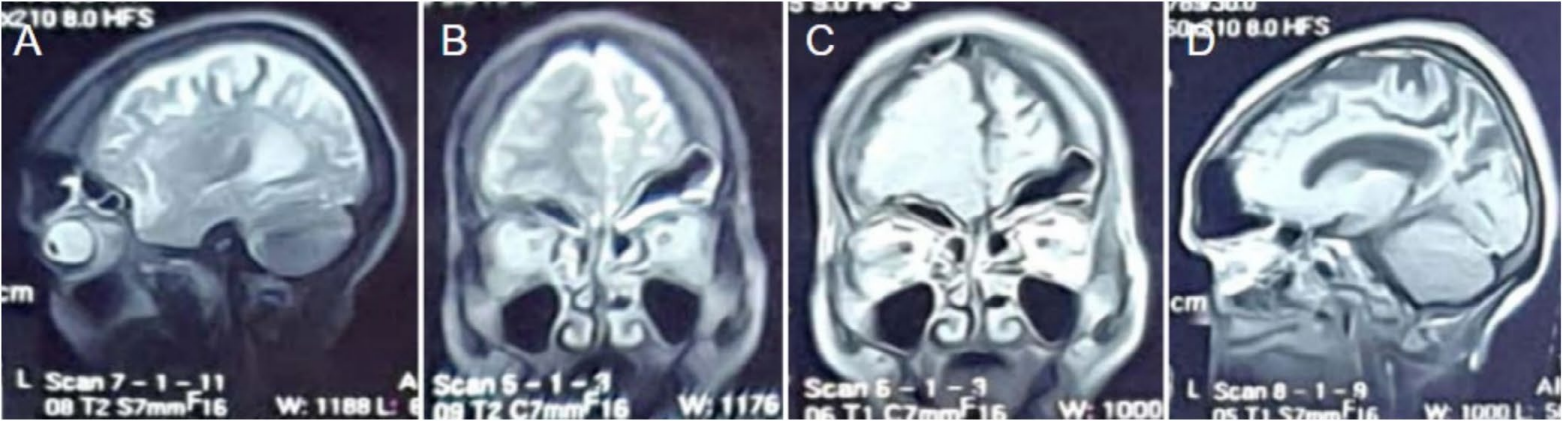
(A) T1 sagittal MRI showing hypertrophy of the left frontal and ethmoid sinuses with left calvarial thickening. (B) T2 coronal MRI demonstrating left hemicerebral atrophy. (C) T1 post‐contrast coronal MRI. (D) T1 post‐contrast sagittal MRI.
